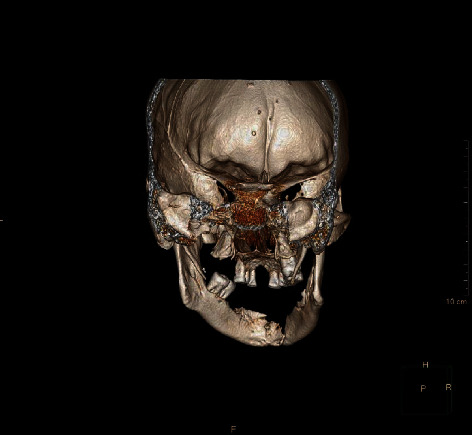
Lingual view of the same CT scan.
